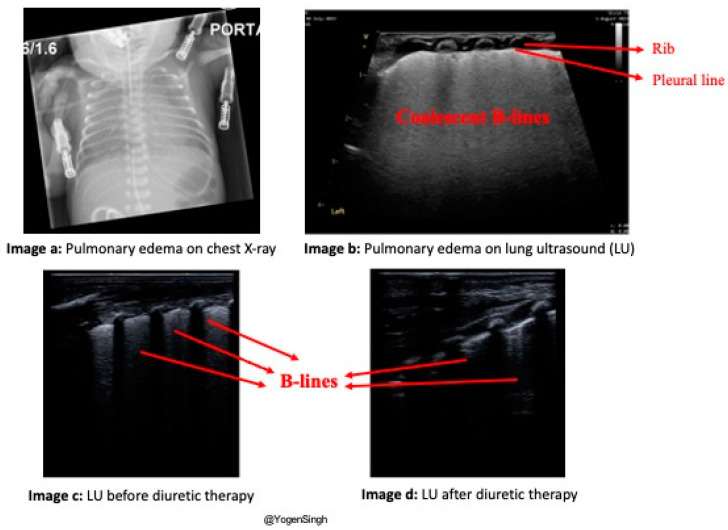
Assessment of pulmonary edema on chest X-ray and lung ultrasound (LU). (a) shows pulmonary edema with increased opacity on the chest X-ray. (b) shows severe pulmonary edema with a white-out appearance on LU. The white-out appearance results from coalescent B-lines, which are echogenic vertical lines below the pleural line, indicating interstitial pulmonary fluid. (c) shows severe pulmonary edema with multiple B-lines before the diuretic therapy. (d) shows fewer B-lines, suggesting decreased pulmonary edema after the diuretic therapy. *LU—lung ultrasound*.
